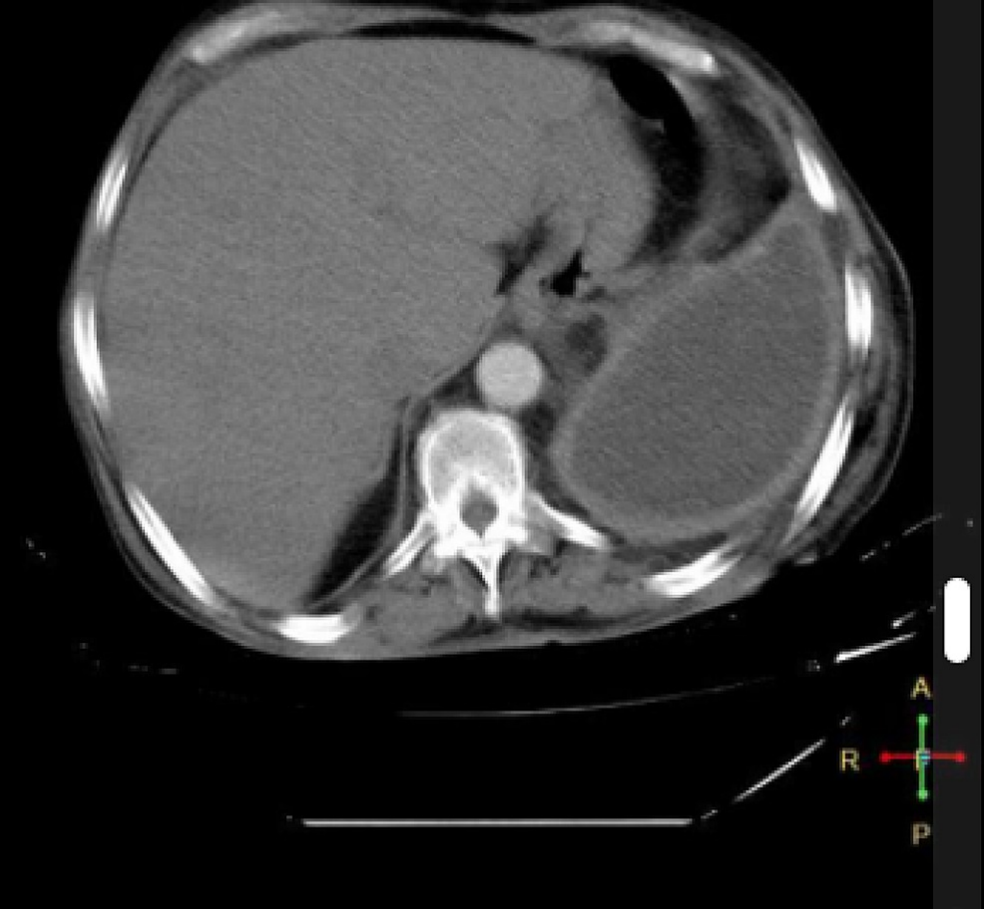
Split pleura sign on CT scan with thick pleural lining. Image courtesy of radiopedia. Creative Commons Attribution-Share Alike 3.0 Unported license. Case courtesy of Dr Ahmed Abdrabou, Radiopaedia.org, rID: 24442
